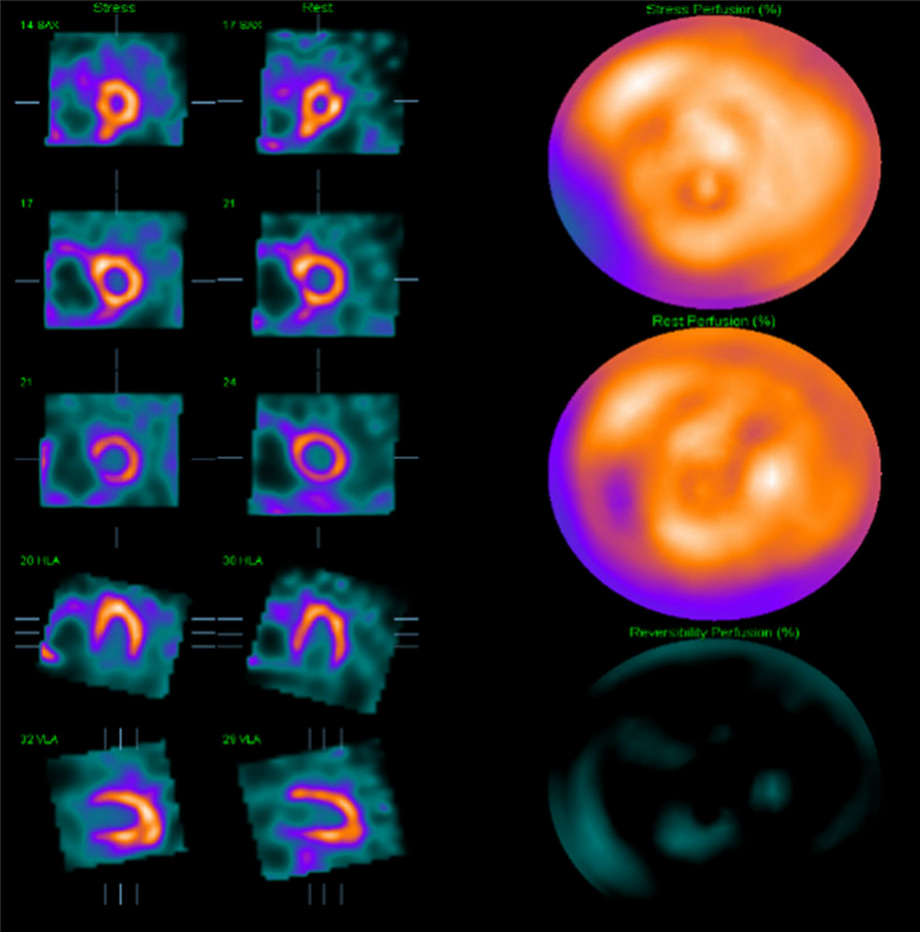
Case 1: Thallium-201 MPS of a 75-year-old man with idiopathic pulmonary fibrosis showing no inducible perfusion abnormality. MPS, myocardial perfusion scintigraphy
